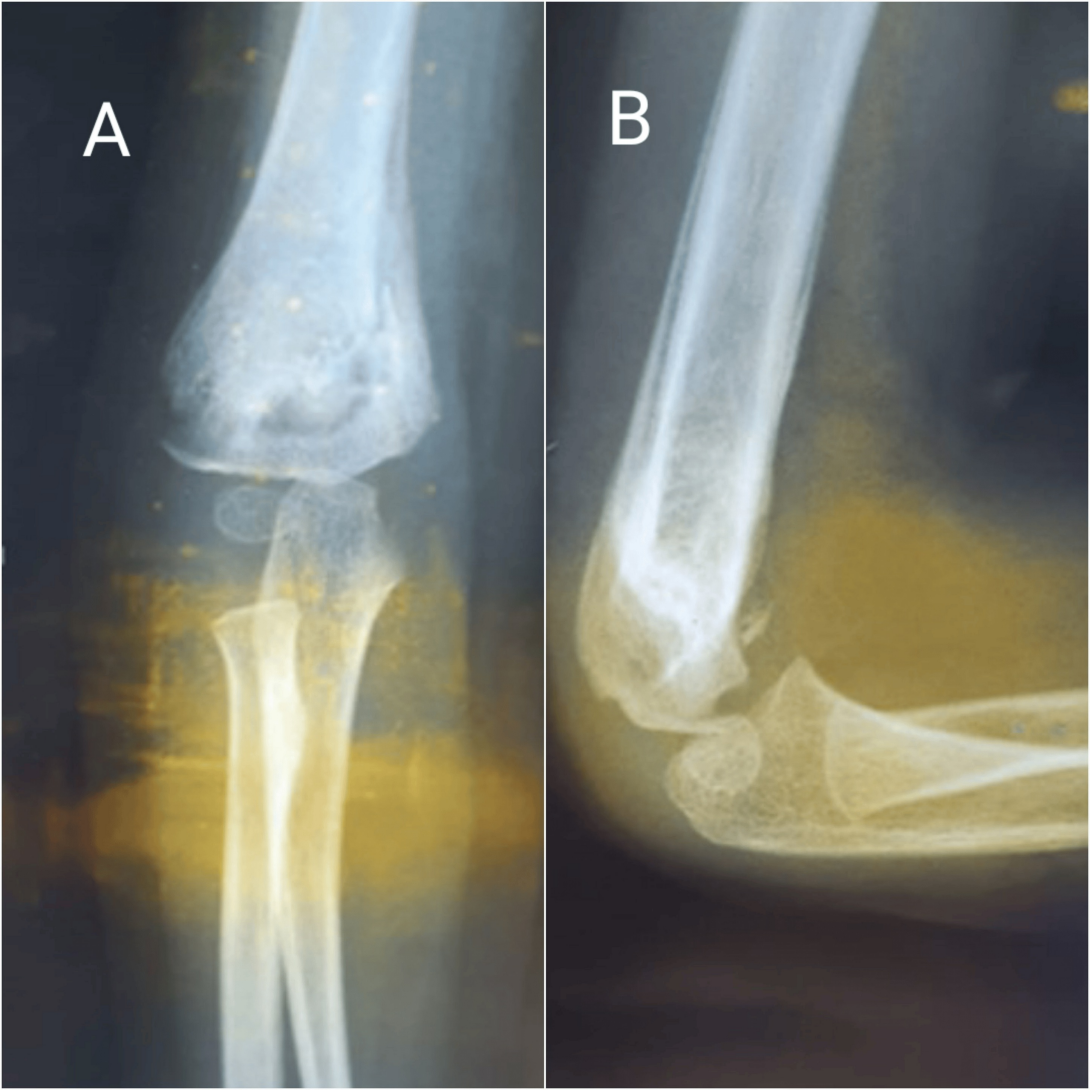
Right elbow X-rays at six-weeks follow-up, following the removal of the Kirschner wires A: Anteroposterior view. B: Lateral view
